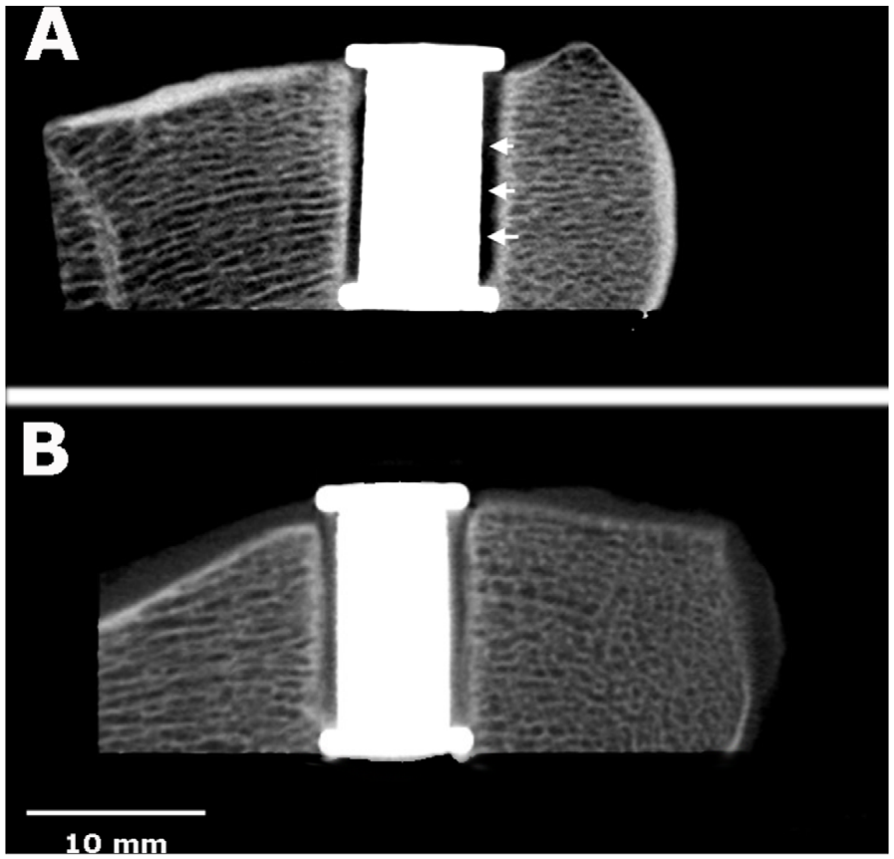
Images of Dyna CT results between control and mBMP coated implants. A: Control, DynaCT demonstrating that gap between the implant and host bone was still visible (white arrows). B: The gap between mBMP coated HAP-implant and host bone was filled with high density mineralized tissue.
